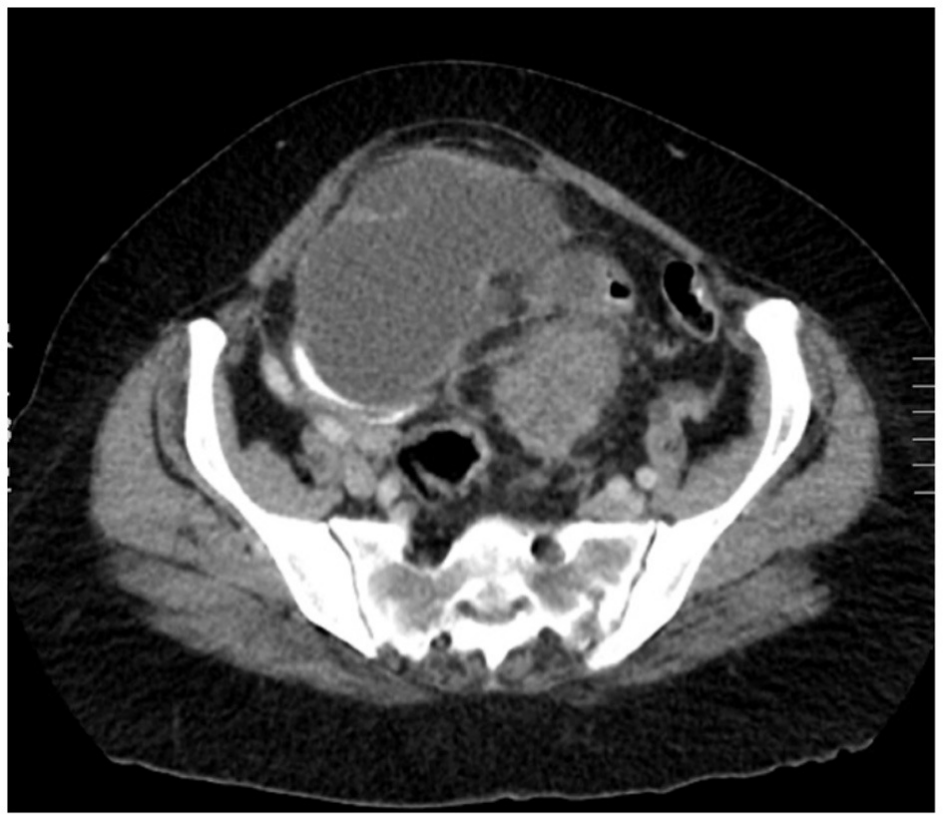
Axial CT with mass measuring ~11 cm × 10 cm.
